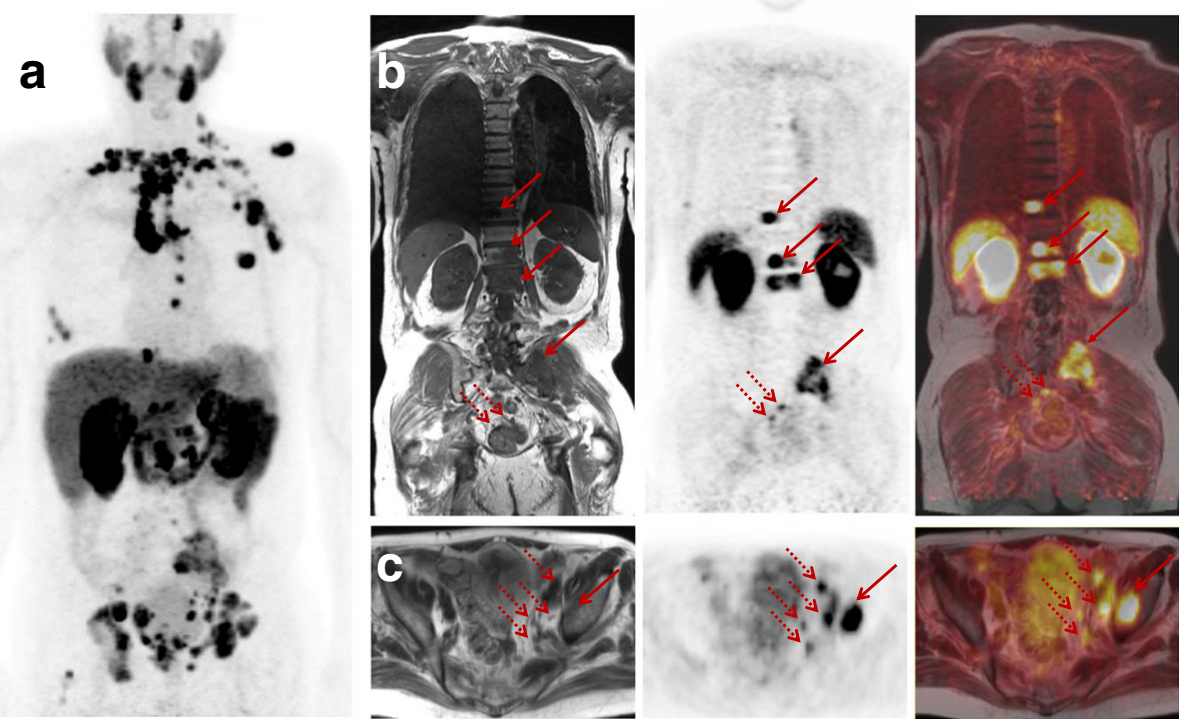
PET/MR imaging in a mCRPC patient (PSA 392 ng/ml) using EuE-k-^18^F-FBOA (1) demonstrates intensive PSMA-ligand uptake in multiple bone (arrows) and tiny subcentimeter lymph node (dotted arrows) metastases. Maximum intensity projection (a) shows distribution of disease. Sets b and c shows coronal and axial MR (T1 and T2 haste), PET and fused images with arrows and dotted arrows representing bone and lymph node metastases, respectively
